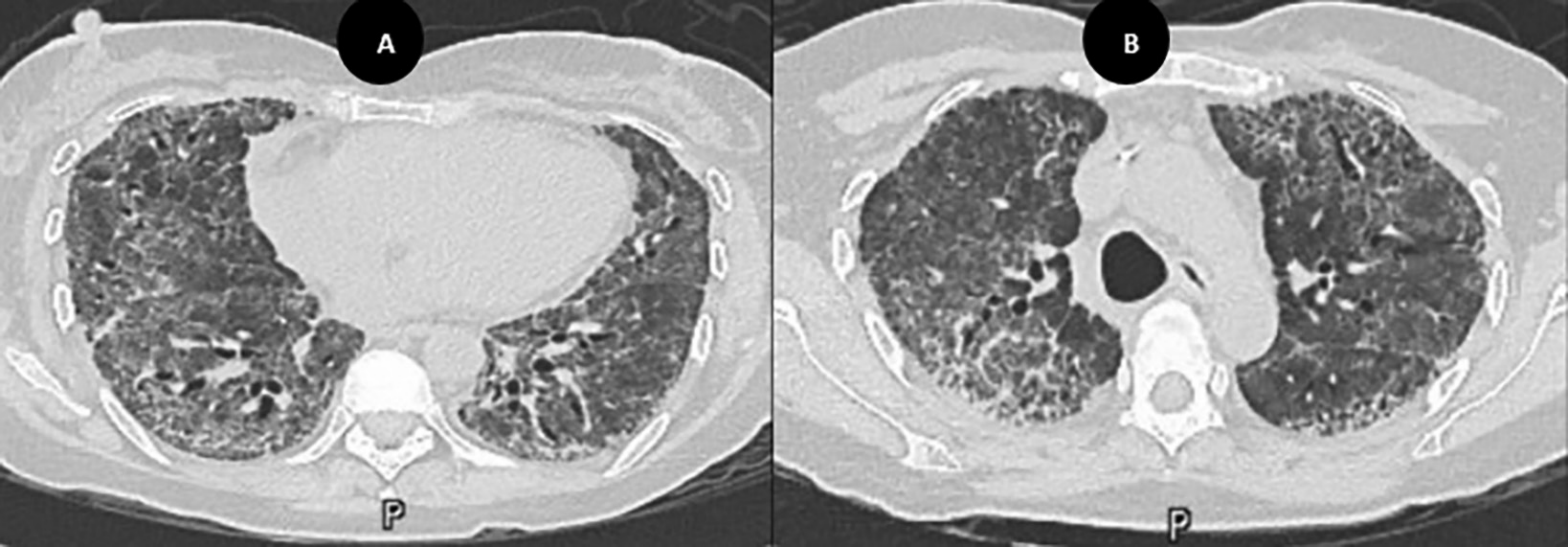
(A, B) Follow‐up HRCT performed 4 weeks after admission demonstrates interval improvement in the widespread ground‐glass opacities, along with evolving fibrotic changes, including interlobular septal thickening and traction bronchiectasis.
